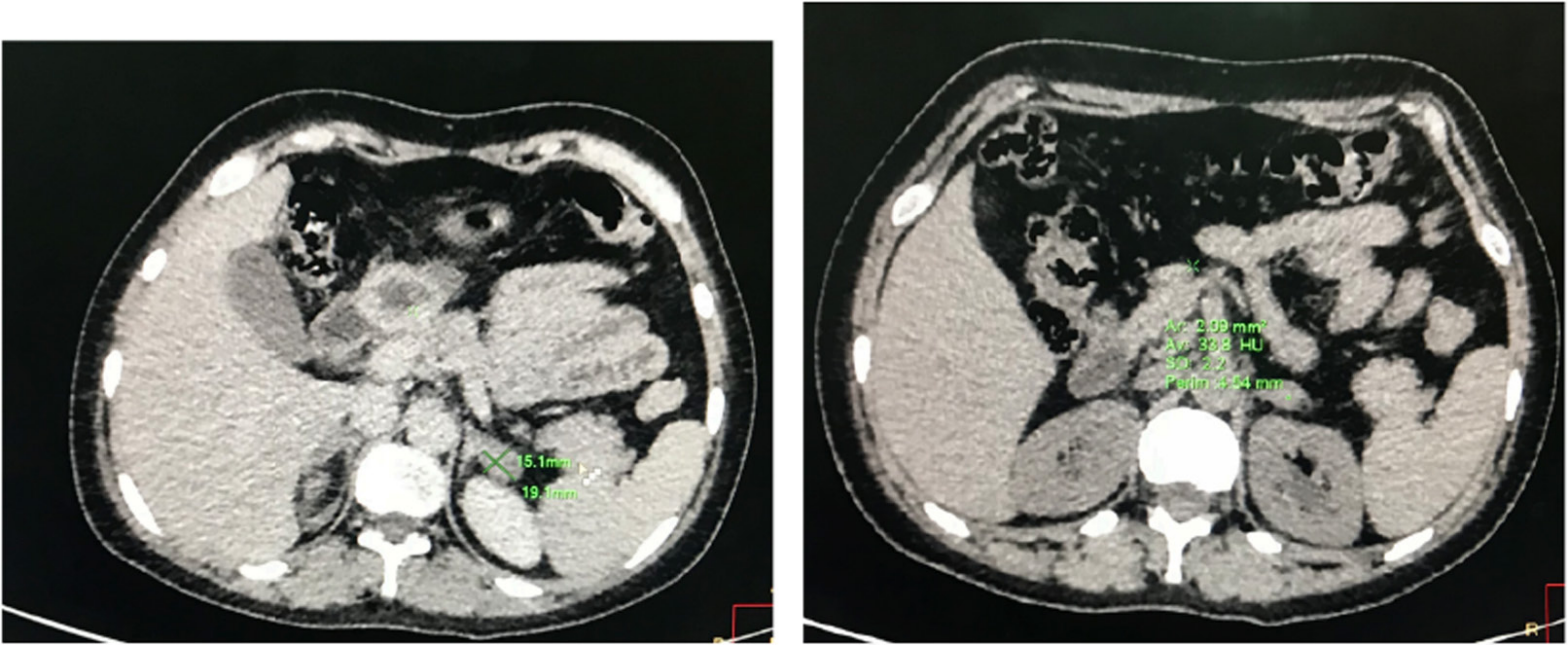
Axial non‐contrast CT image showing bulky left adrenal with a well‐defined nodular lesion in the lateral limb. No evidence of calcifications or fat attenuating areas within
